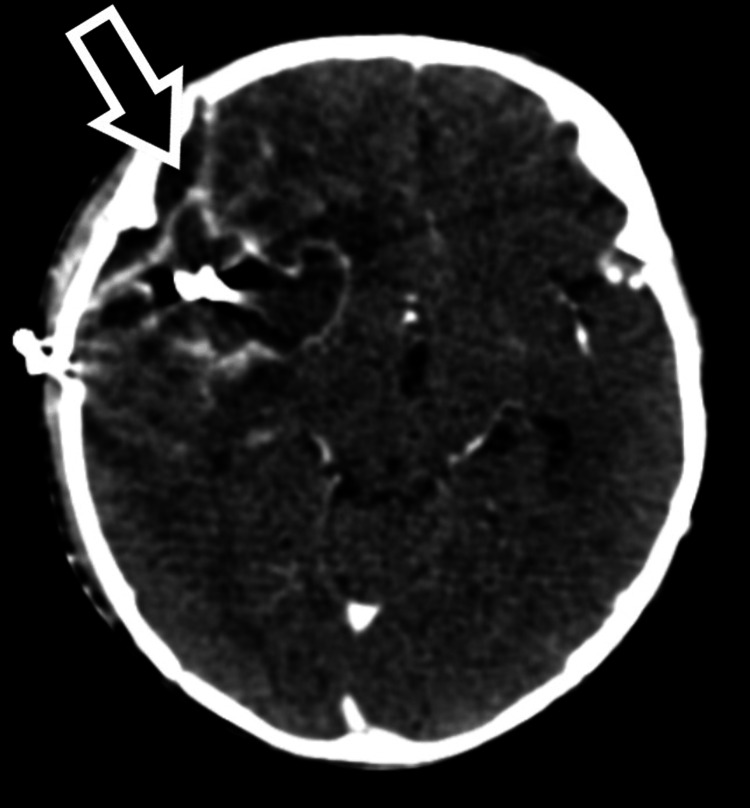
Post-operative computed tomography of the brain (plain axial section) showing resolving intraparenchymal hemorrhage with reduced midline shift and cerebral edema in the right frontotemporal lobe with metallic surgical clip in situ (black arrow), suggesting right MCA aneurysm clipping. MCA, middle cerebral artery
